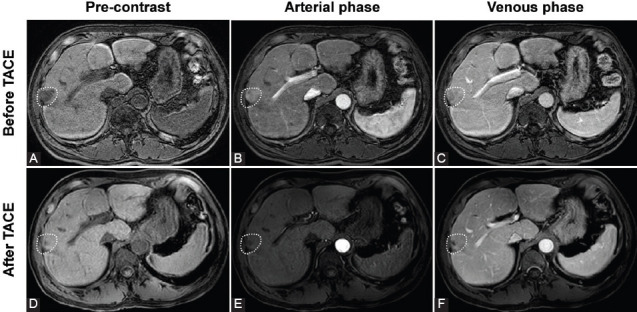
Magnetic resonance images of a successful spherical, calibrated, biodegradable, radiopaque microspheres (SCBRM)-based TACE treatment in a representative patient. (A-C) Baseline imaging of the patient. (A) Pre-contrast T1-weighted image showed the tumor located at the right hepatic lobe with a size of about 2.6 cm (white circle). (B) Arterial phase T1-weighted image showed arterial enhancement. (C) Venous phase T1-weighted image showed the washout. (D-F) After the SCBRM-based transarterial chemoembolization (TACE) treatment. (D) Pre-contrast T1-weight image showed tumor shrinkage. (E) Arterial phase T1-weighted image showed no arterial enhancement. (F) Venous phase T1-weighted image showed no washout enhancement.
